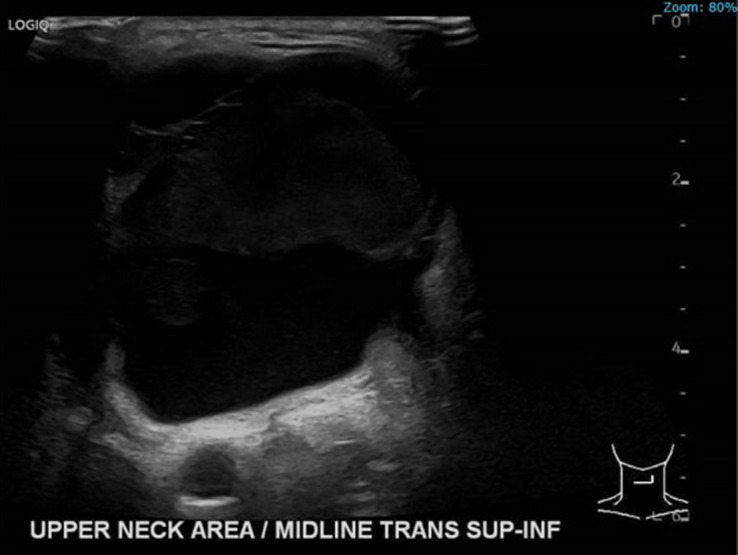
Neck ultrasonography showing thyroglossal duct cyst prior to ethanol ablation.
